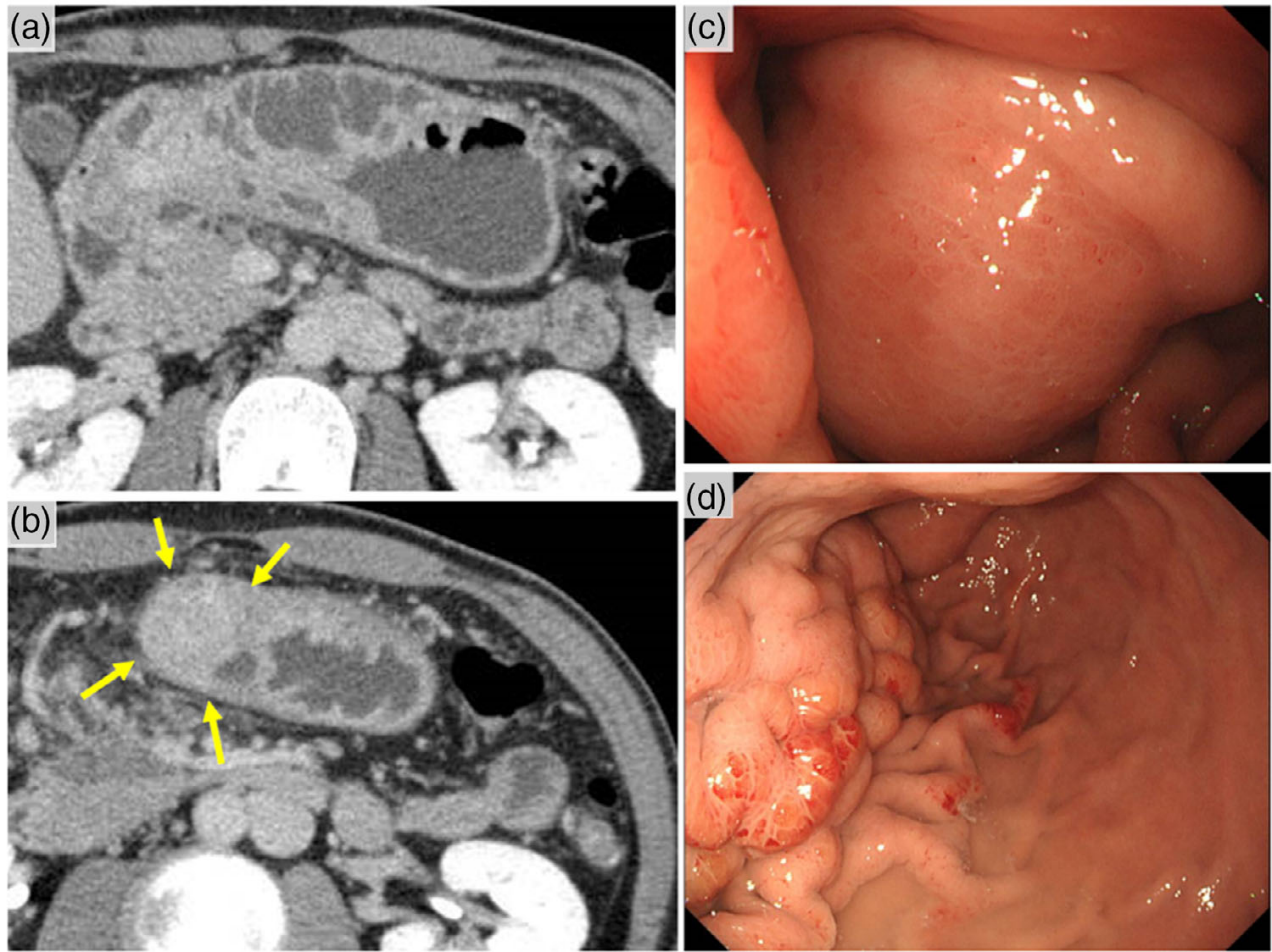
Twenty‐three months after the initial onset, he was hospitalized due to severe vomiting, preventing food intake. Contrast‐enhanced abdominal computed tomography revealed a massive gastric wall thickening, and gastric intramural lesion (arrows) (a,b) and gastroscopy revealed edematous gastric mucosa with edematous stenosis (c,d).
